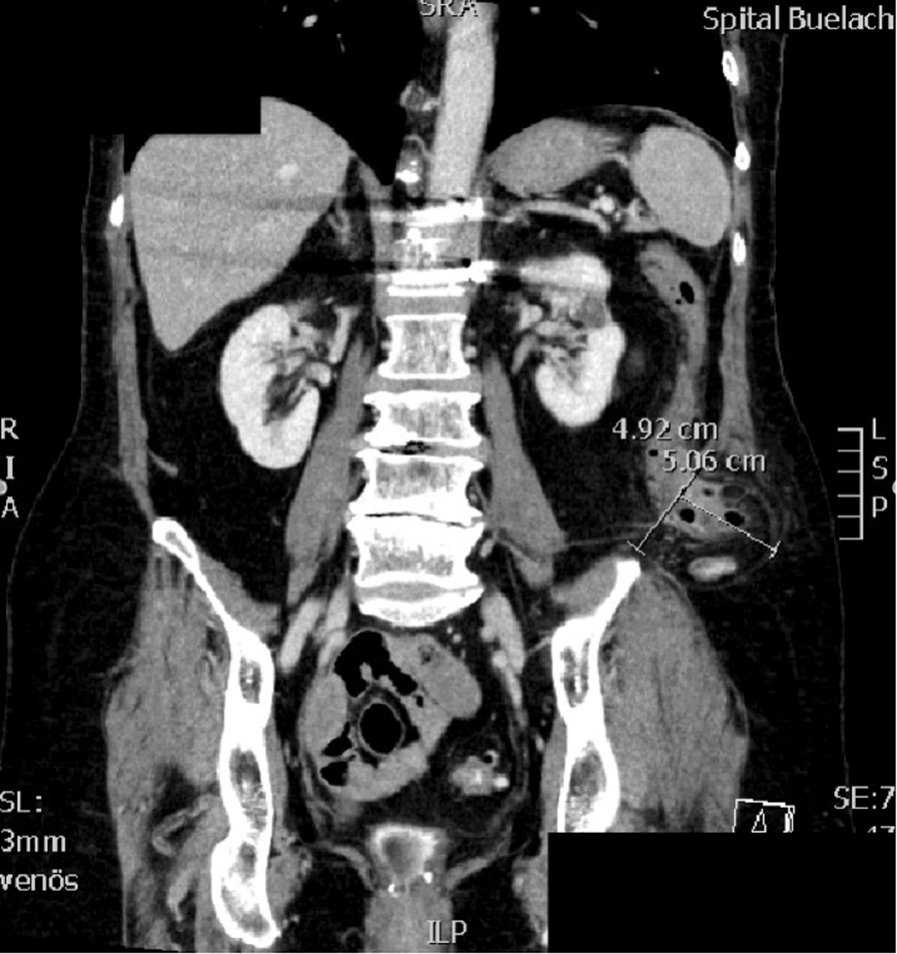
Abdominal CT scan, showing perforated sigmoid colon in a left lumbar hernia.
